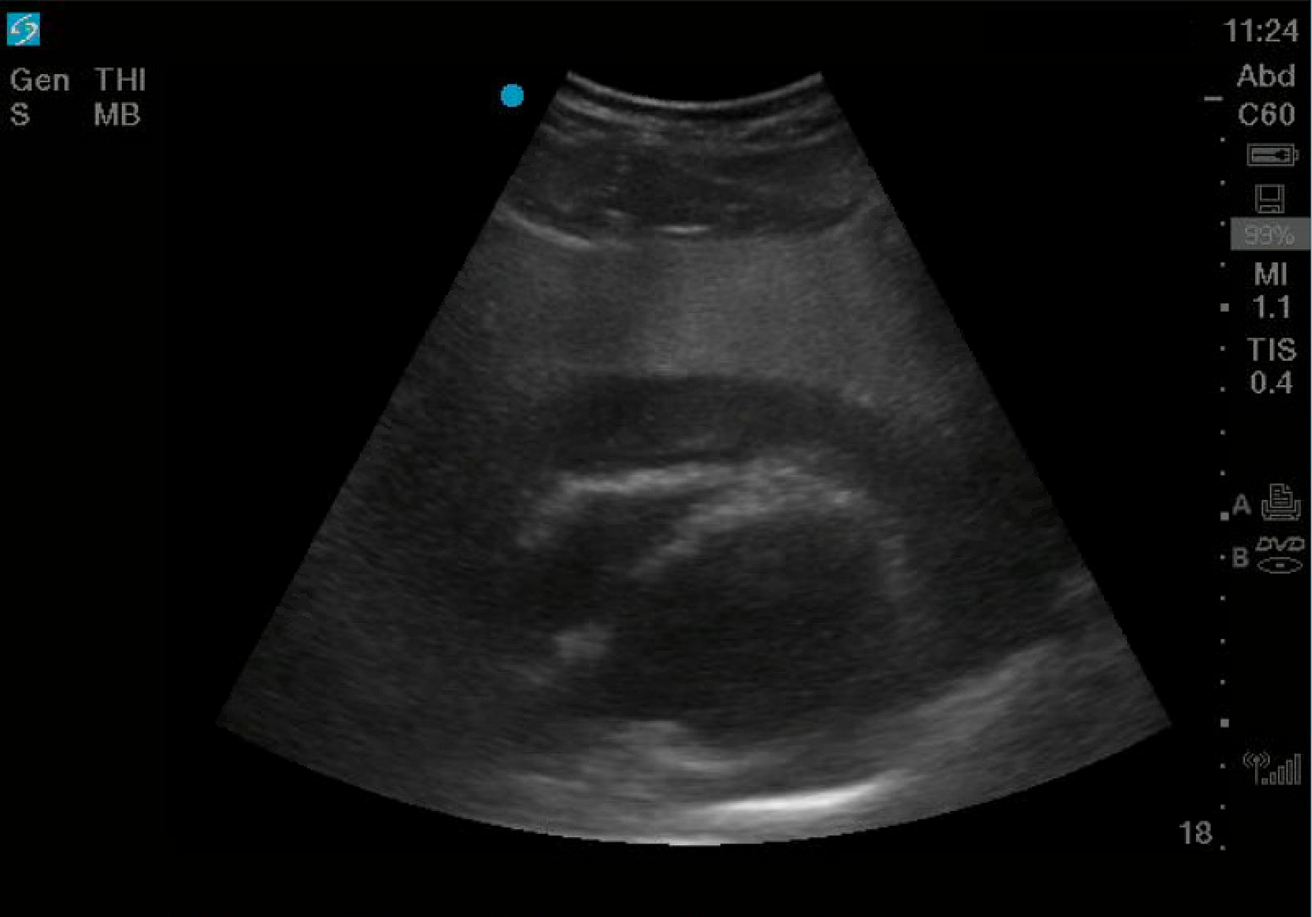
Ultrasound demonstrating a large pericardial effusion
